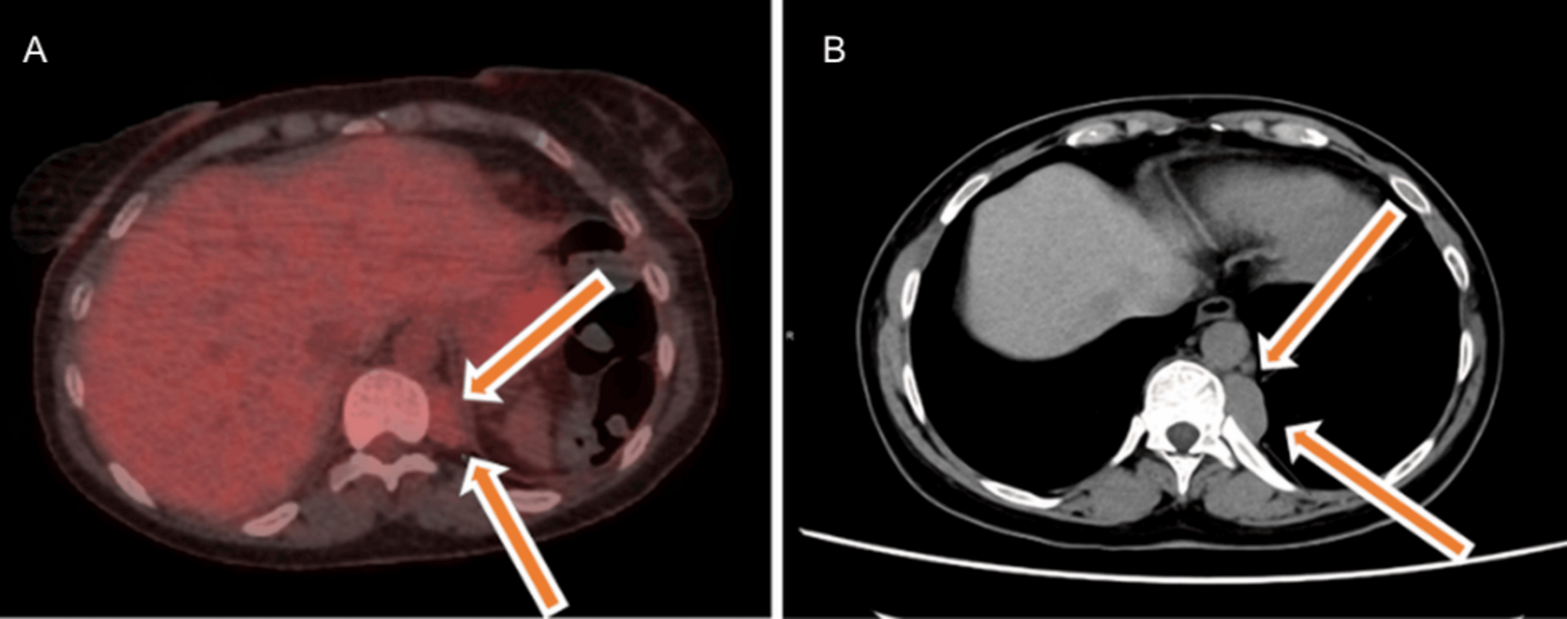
(A, B) Positron emission tomography-computed tomography (PET-CT) and CT images demonstrating the paravertebral lesions (arrows).
